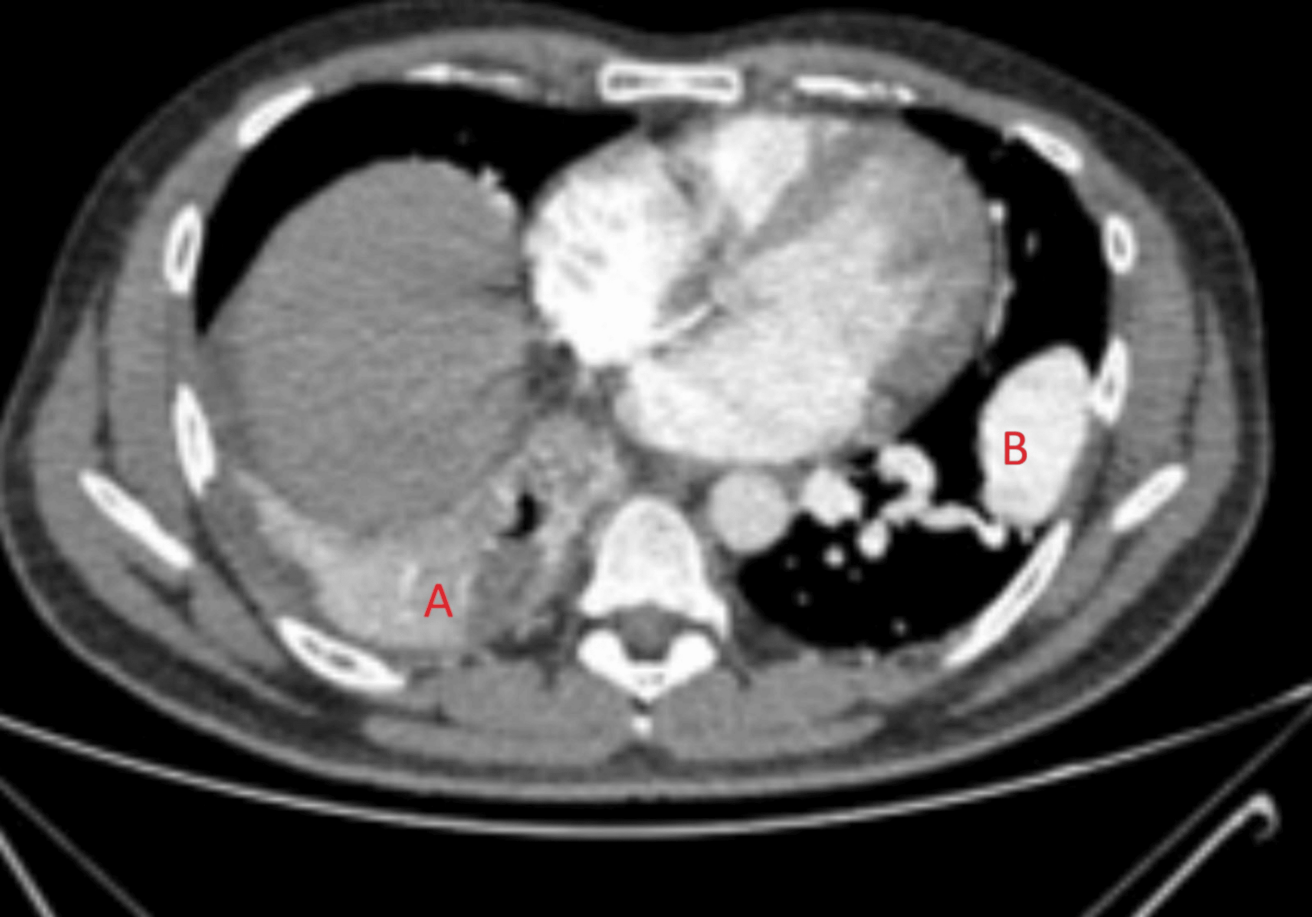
CT thorax with contrast (mediastinal window) A- Collapse consolidative changes with air bronchogram and focal cavitatory lesion (3.2 x 2.4 cm) in right lower lobe, suggestive of infective etiology. B- Homogenously enhancing, well circumscribed, juxtafissural soft tissue density nodule in left lung with feeding artery as segmental branch from left lower lobar artery and draining vein as left inferior pulmonary vein. Features are suggestive of pulmonary arteriovenous malformation with nidus.
